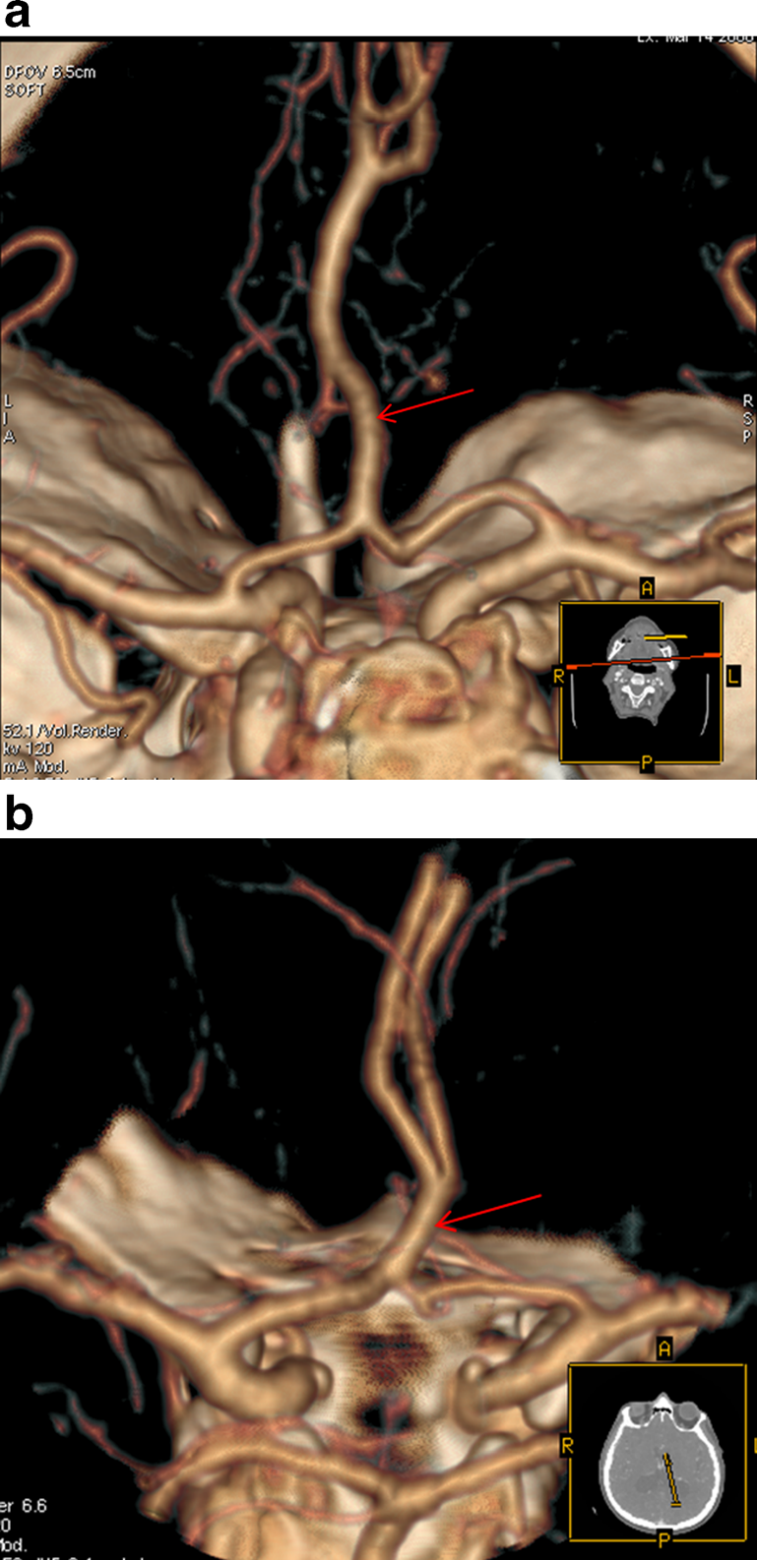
a CTA (VRT 3D reconstructions) reveals the presence of an unpaired ACA (conventional type, long segment) (*red arrow*). b CTA (VRT 3D reconstructions) shows an unpaired ACA (conventional type, short segment) (*red arrow*)
